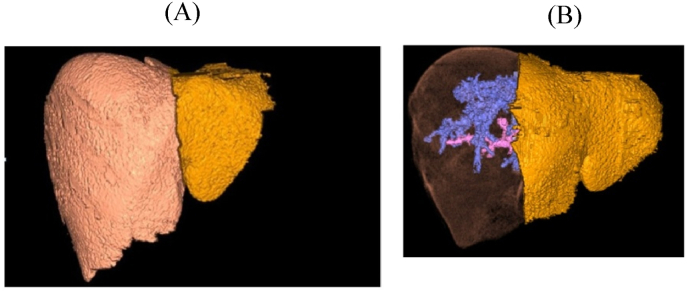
CT with hepatic protocol and 3d imaging reconstruction showing: A: Left lateral graft(yellow) with expected GRWR of 2.2(chosen). B: Left lobe graft(yellow) with expected GRWR of 4.9(Excluded to avoid LFSG). (For interpretation of the references to colour in this figure legend, the reader is referred to the Web version of this article.)
